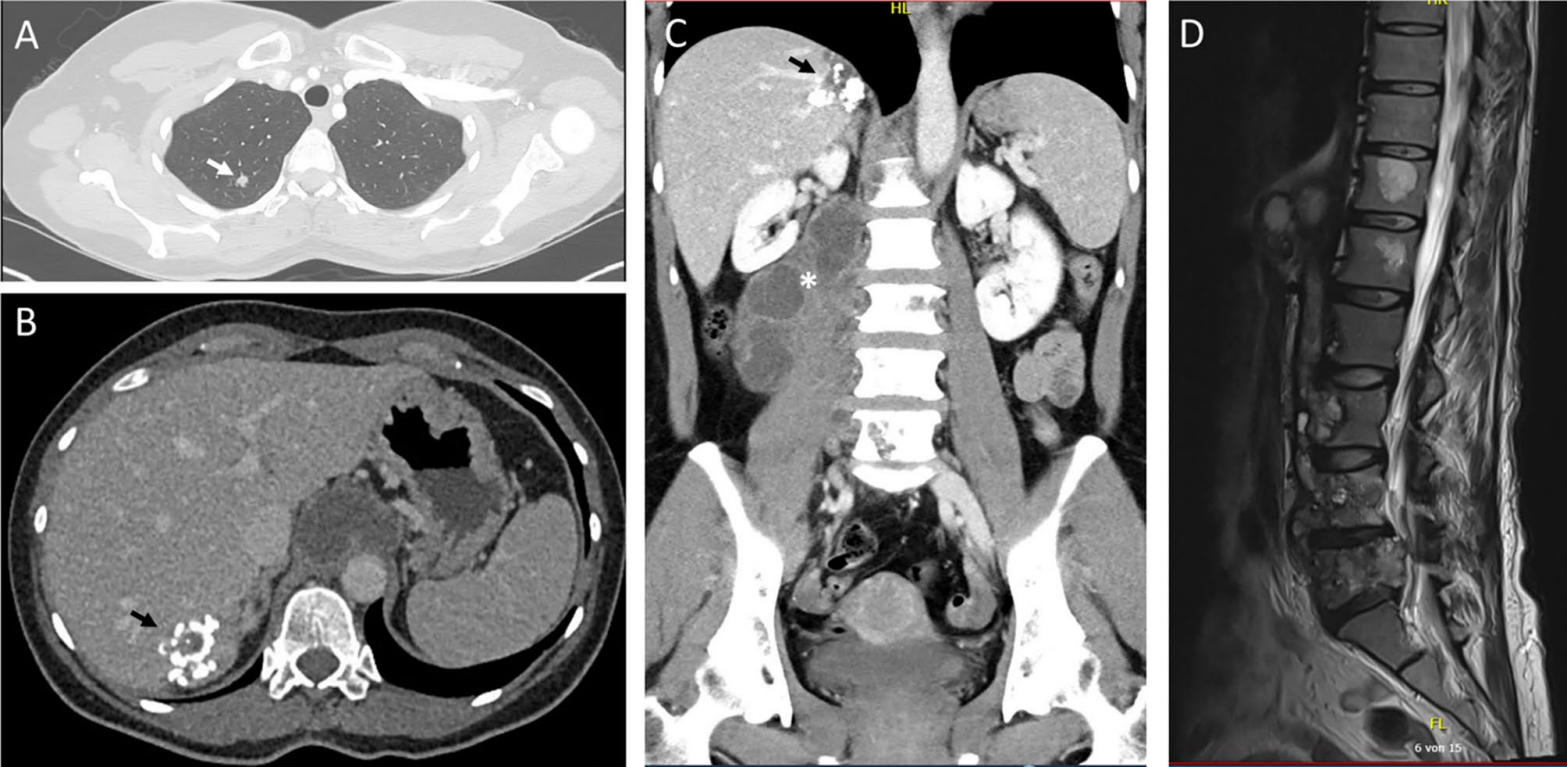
A, B Axial CT images at initial presentation show dense pulmonary nodules (white arrow) and a ring-shaped hepatic calcification (black arrow). C Coronal CT reconstruction depicts the hepatic lesion and an approximately 11 × 4 cm hypodense, multilobulated mass extending from the interaortocaval region along the psoas muscle to the aortic hiatus (asterisk), with osteolysis of lumbar vertebrae 3–5. D MRI after eight weeks of anti-tuberculosis therapy reveals progressive osteodestruction with a small polycystic or honeycomb appearance and inflammation involving the ventral epidural space
